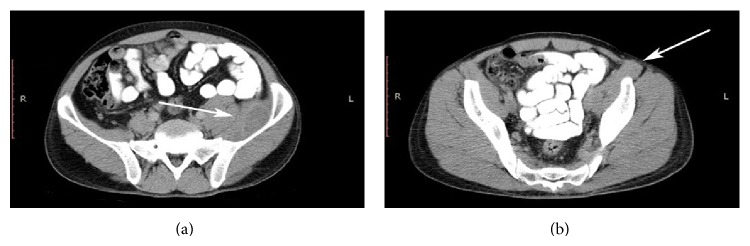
Repeat CT sections after 3 weeks showed significant reduction of hematoma. White arrows in the pictures highlight left iliopsoas hematomas reduction and the reduction of its inguinal extension.
